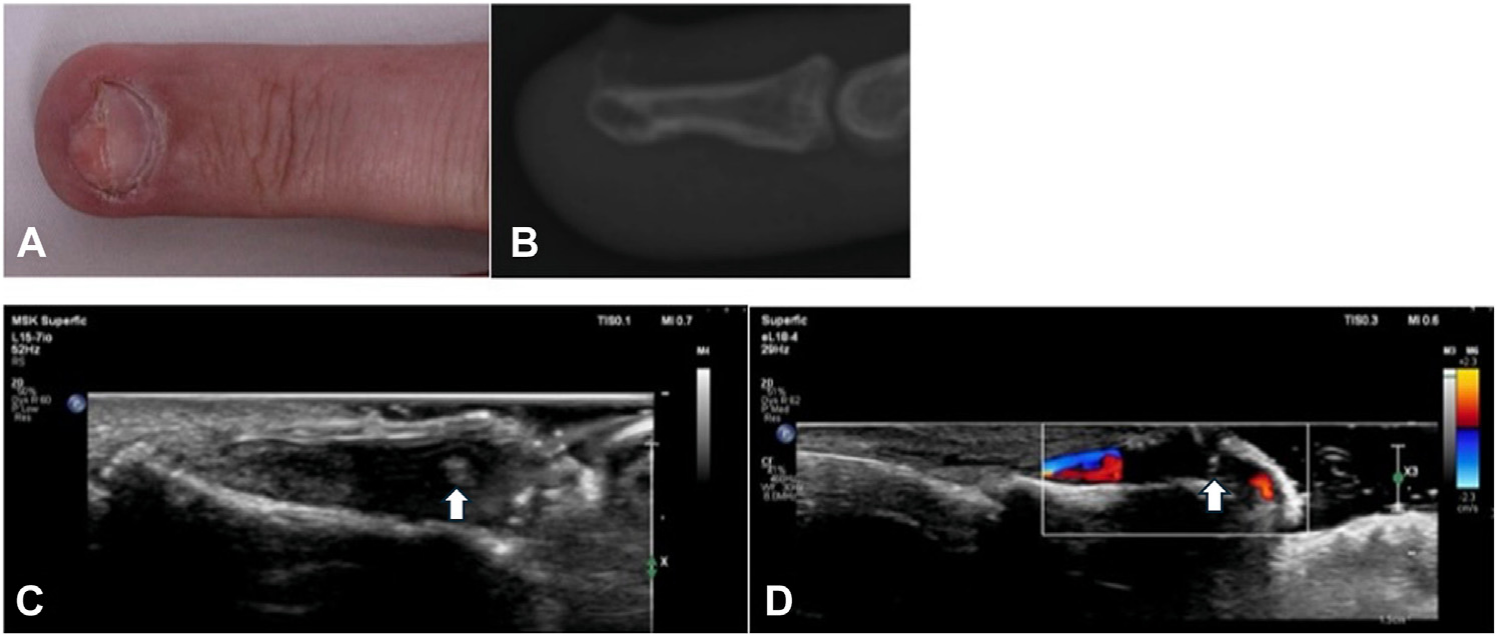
A, Initial clinical exam with a 6 mm skin-colored exophytic subungual papule on the distal tip of the right fourth finger without overlying epidermal changes. B, X-ray showed a 2 mm peripherally calcified low-density lesion overlying the dorsal tuft of the fourth digit distal phalanx. C and D, Ultrasound images of the right fourth digit using a high-frequency probe (*arrow* pointing to osteoma cutis). C, Nail bed appears hypoechoic with small round hyperechoic foci, consistent with calcification without any bony defect of the underlying distal phalanx. D, Doppler ultrasound demonstrating normal vascular flow without hypervascularity of the lesion.
